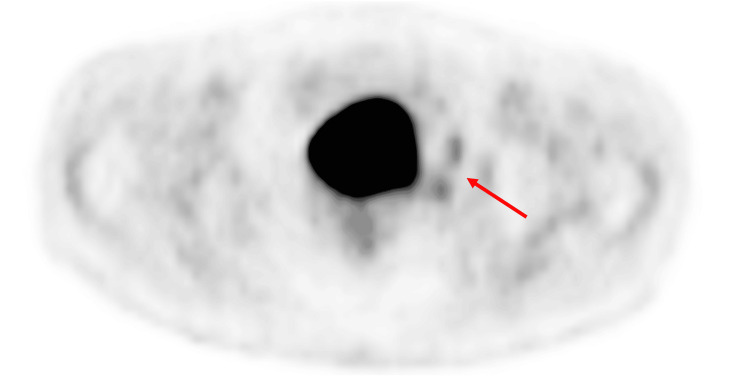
A more cranial positron emission tomography/computed tomography slice demonstrates a hint of focal uptake suspected to be the neck of the diverticulum extending from the bladder.
